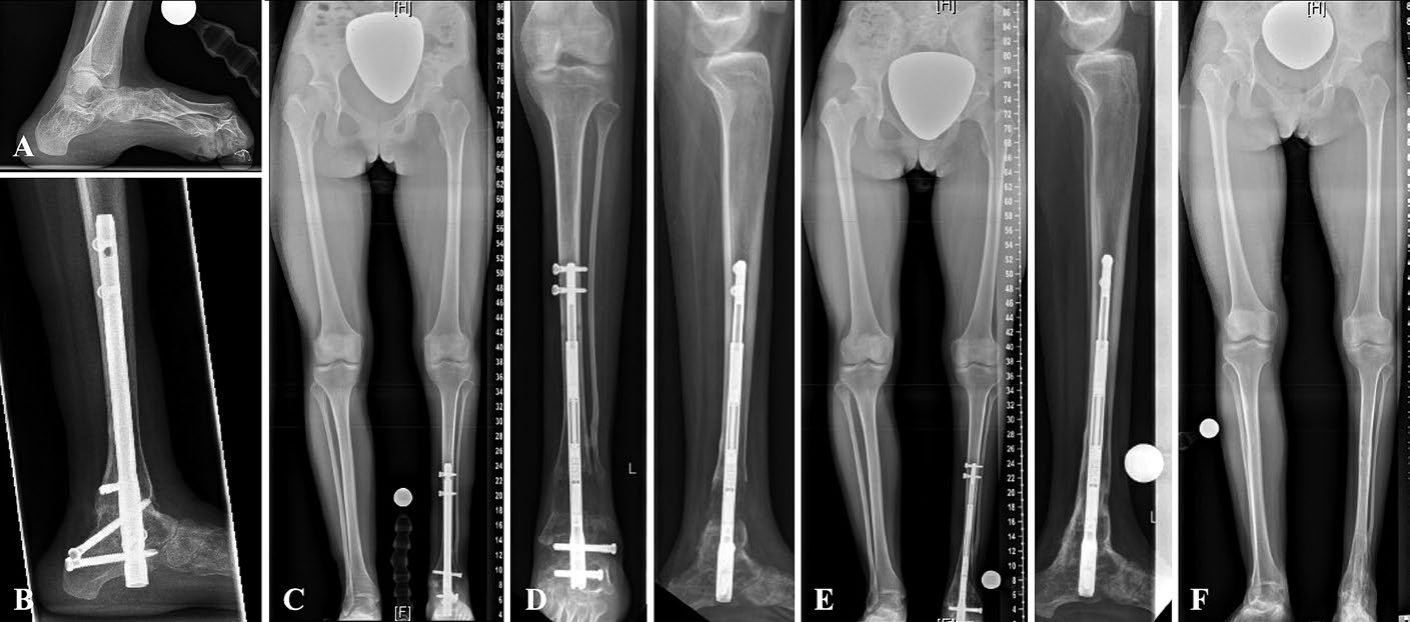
A—Patient No. 3 with pes calcaneus and leg shortening due to caudal regression syndrome. B—Prior to lengthening the foot deformity was corrected by ankle and subtalar arthrodesis using a retrograde nail. C—After ankle and hindfoot fusion the residual LLD measured 38 mm. D—Tibial distraction osteogenesis of 31 mm using a retrograde magnetically driven ILN. E—Postoperative long standing radiograph after full consolidation. F—Postoperative long standing radiograph after ILN removal 26 months postoperatively showing intended residual LLD of 7 mm.
